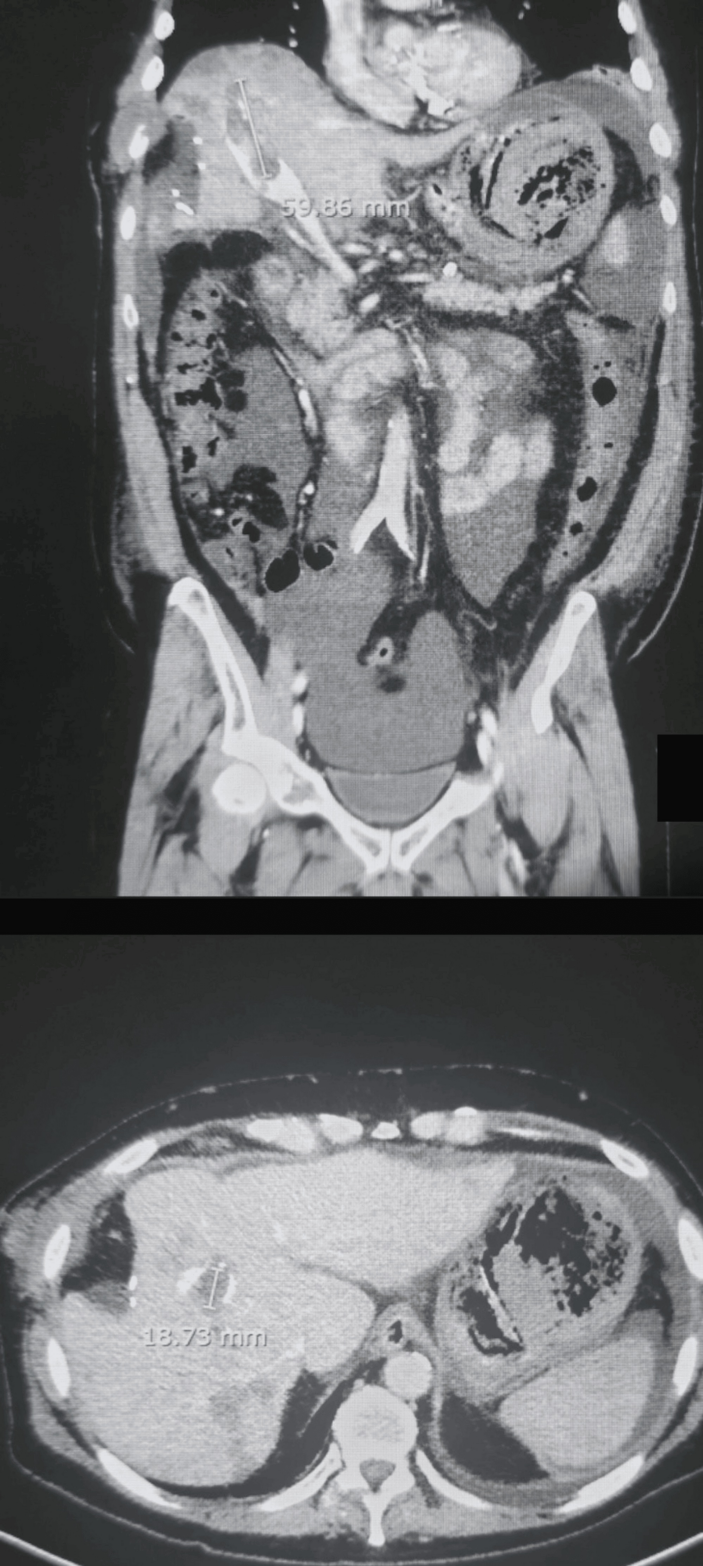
CT images showing hepatic metastasis and tumor-associated right portal vein thrombosis. CT, computed tomography
